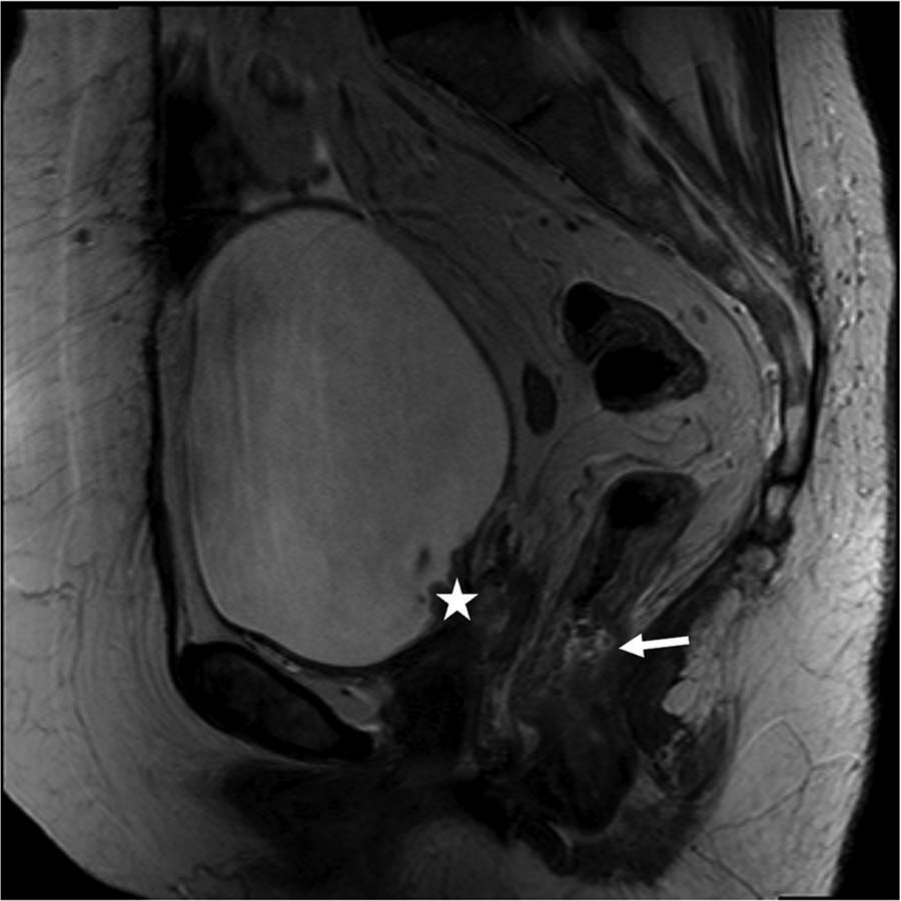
Rectal MRI, sagittal view. Star marks vaginal cuff mass with bladder invasion; arrow marks rectal tumor.
